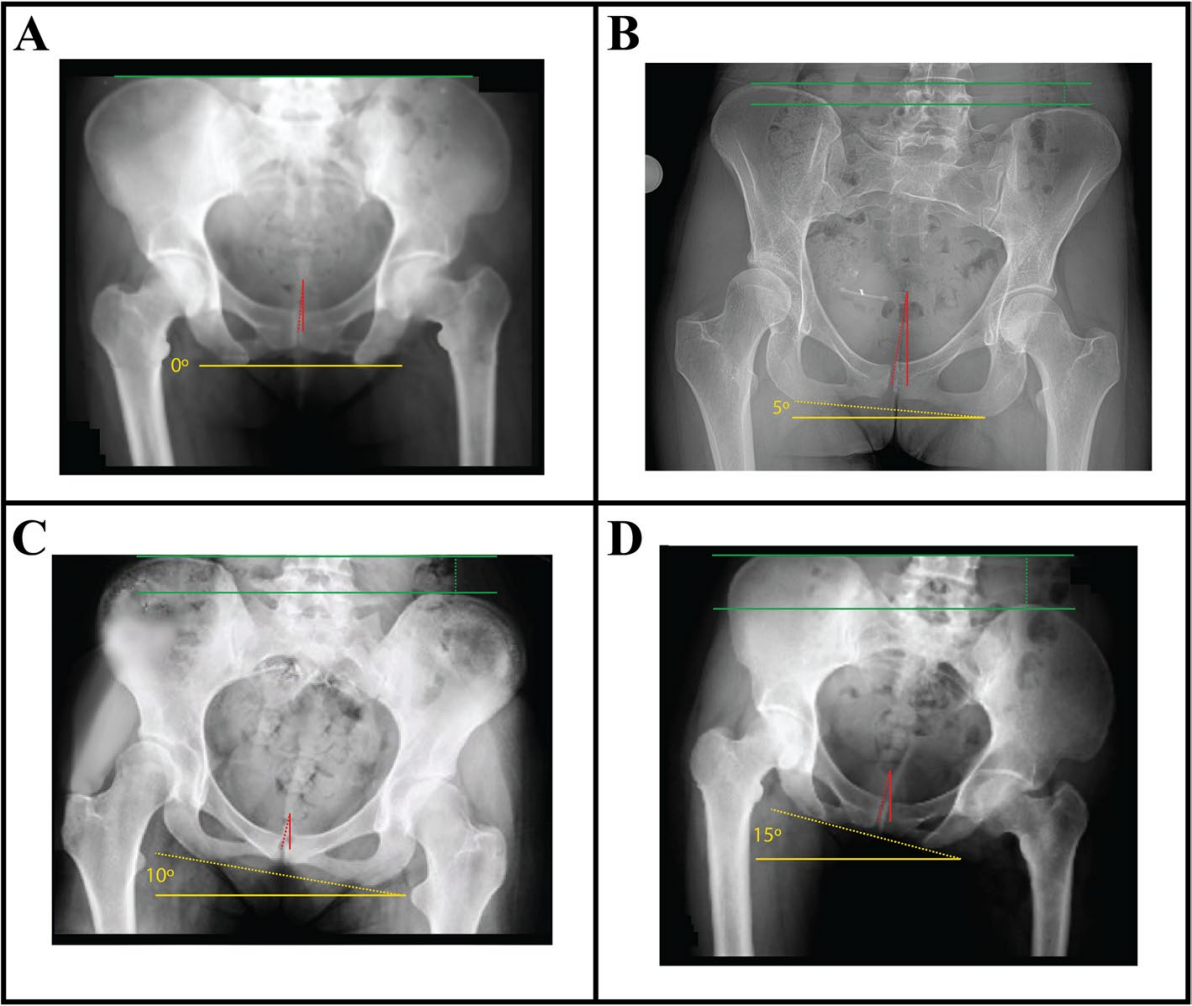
A An AP pelvic radiograph of a patient with a level pelvis is shown. Notice the relative bilateral symmetry in the size and shape of the obturator foramina, the iliac wings, the ischial spines, and the ischial tuberosities. This symmetry, viewed in context of the relative vertical alignment of coccyx and the midline of the pubic symphysis gives us an overall impression of a lack of PO. B A PO of 5° is seen in this radiograph. Notice the bilaterally asymmetric heights of the iliac crests and the relatively increased vertical malignment of the tip of the coccyx and the midline of the pubis compared to the neutral pelvis. C At a PO of 10°, the asymmetry between the bilateral obturator foramina and the heights of the iliac crest become much more profound. D Demonstrating even greater postural asymmetry, this radiograph shows a PO of 15°
